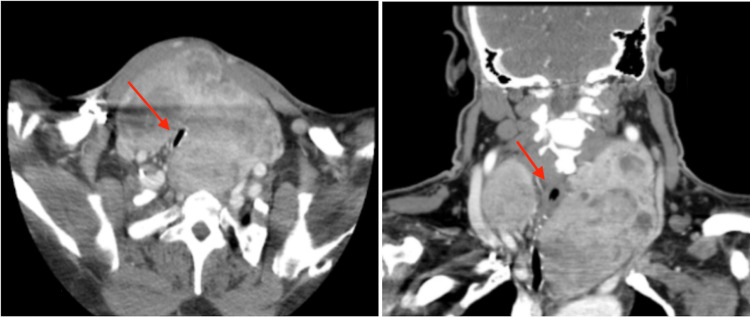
A CT scan of the patient’s neck in axial view (left) and coronal view (right). A CT scan of the neck was obtained with contrast. The compressed and deviated airway is shown by the red arrows.
